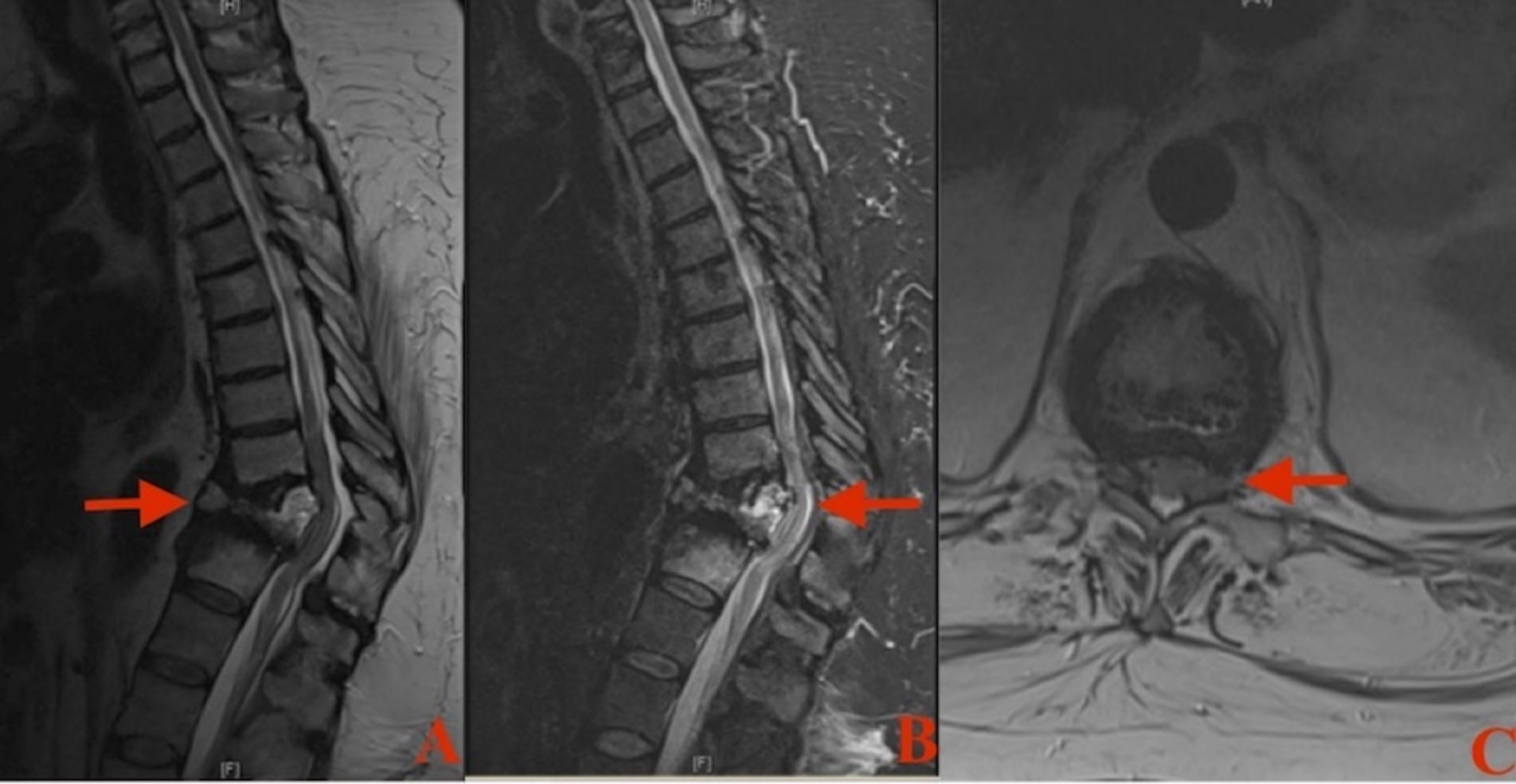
Magnetic resonance imaging (MRI) of the thoracic spine A: Sagittal T2-weighted image demonstrating a T11 burst fracture (arrow) with posterior bony retropulsion and spinal cord compression. B: Sagittal T2-Short Tau Inversion Recovery (STIR) demonstrating areas of hyperintensity within the T11 vertebral body consistent with acute inflammation and intramedullary hyperintensity at T11/T12 consistent with myelomalacia (arrow). C: Axial T2-weighted image at T11/T12 intervertebral disc demonstrating severe spinal canal stenosis with cord compression anteriorly (arrow).
